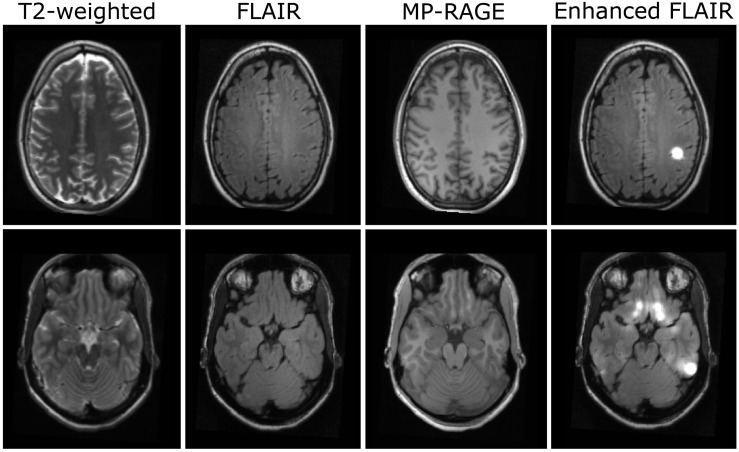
Examples for artifacts in datasets of two healthy subjects (rows). From left to right: T2-weighted, FLAIR-, MP- RAGE-, and enhanced FLAIR-datasets.
